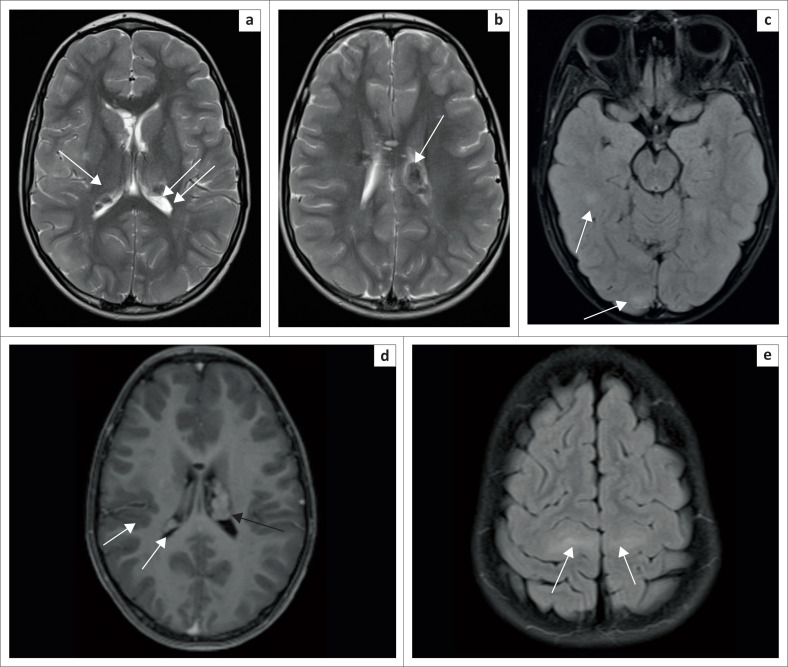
(a) Eleven-year-old male. T2W axial demonstrates numerous hypointense subependymal nodules (white arrows). (b) T2W axial demonstrated large subependymal giant cell astrocytoma (SEGA), with hypointense signal (white arrow). Additional subependymal nodule (black arrow). (c) Fluid inversion axial demonstrates hyperintense tubers (white arrows). (d) Axial T1-weighted precontrast image. Iso-intense subependymal nodule (white arrow). Subependymal giant cell astrocytoma (black arrow). (e) Axial fluid-attenuation inversion recovery (FLAIR) hyperintense white matter radial bands (white arrows).
